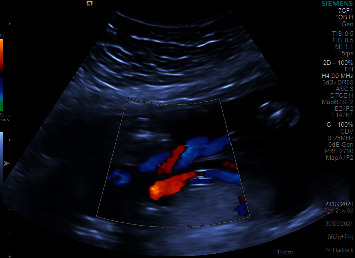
Ultrasound anomaly scan of a fetus at 21 weeks and six days of gestation with ectopia cordis showing that the heart lies completely outside the chest cavity (arrow).
